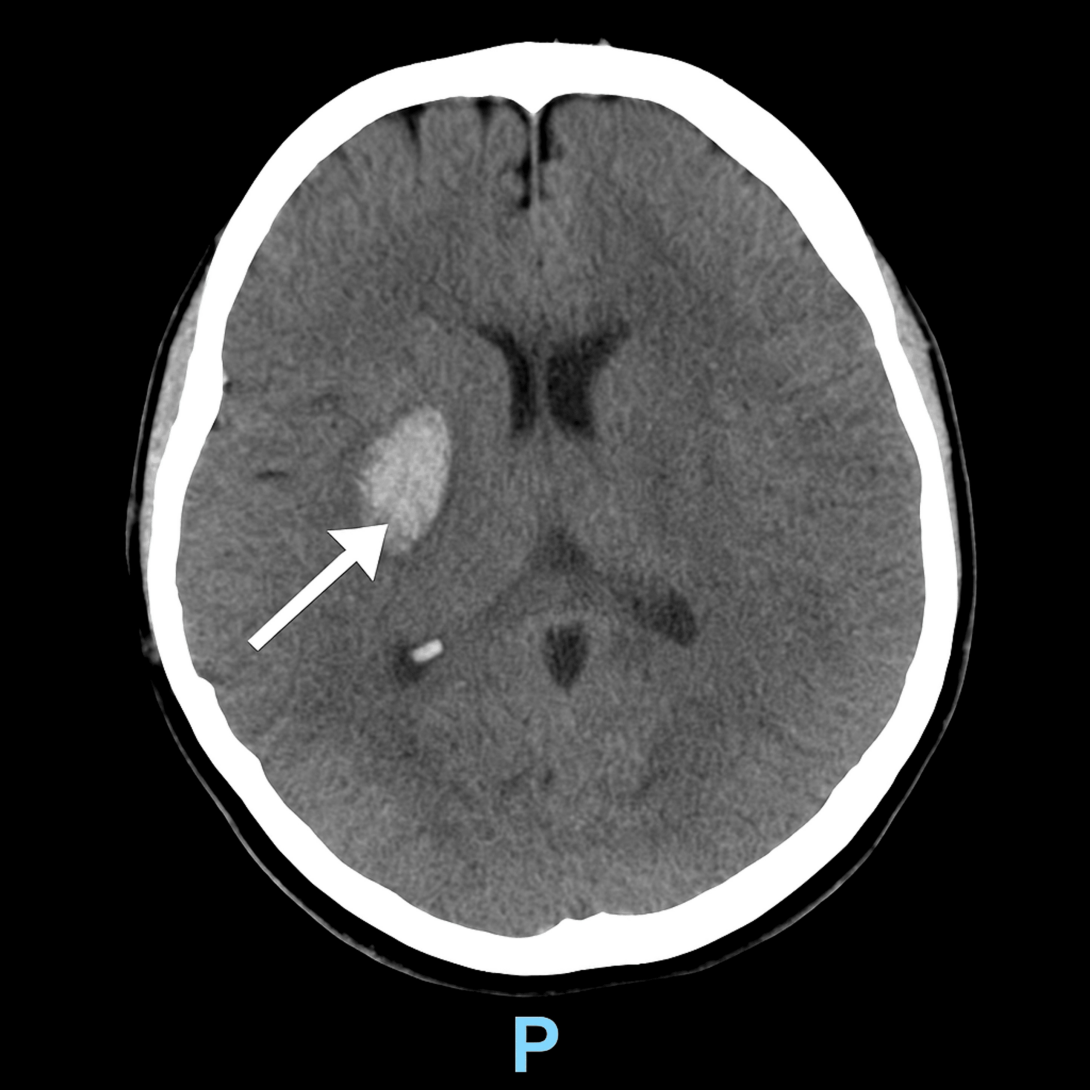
CT scan showing right basal ganglia haematoma
